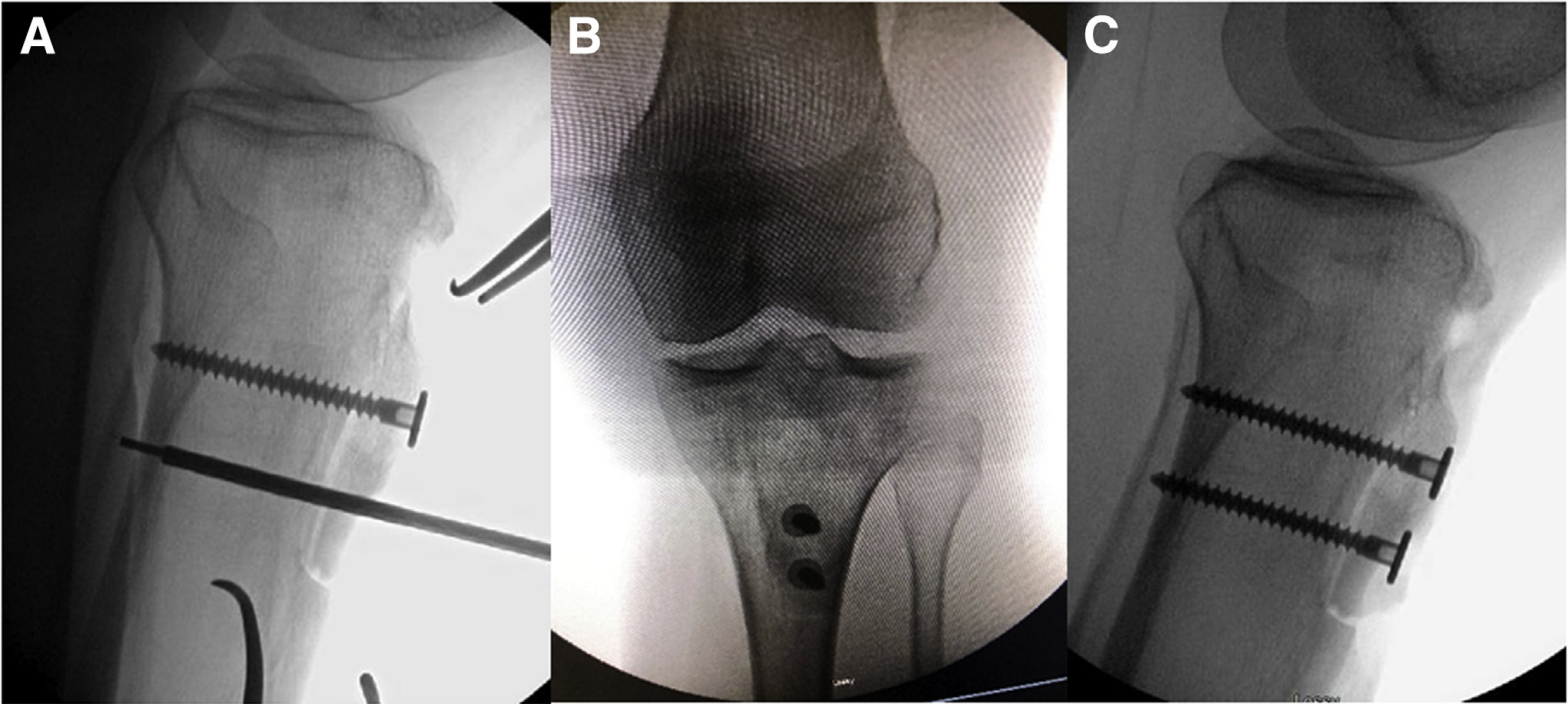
Intraoperative images of X-ray fluoroscopy of a left knee showing tibial tubercle fixation after distalization osteotomy. (A) Overdrilling of the proximal cortices is performed, followed by placement of 2 fully threaded 4.5-mm screws (Arthrex) in an anterior-to-posterior fashion. (B, C) Fluoroscopy is used to again confirm final screw positioning on anteroposterior (B) and lateral (C) views.
